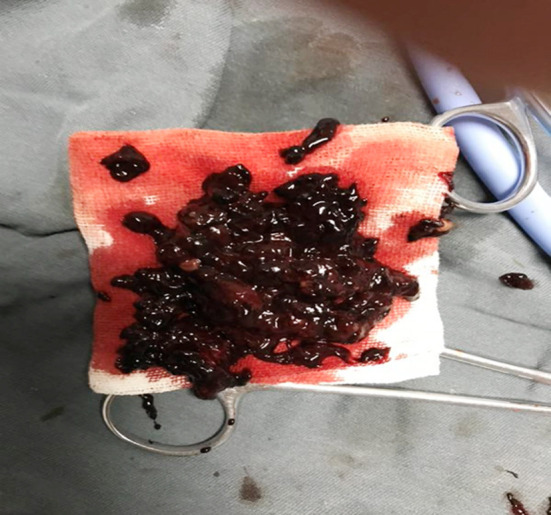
Ultrasound-guided vacuum-assisted biopsy of hematoma.
